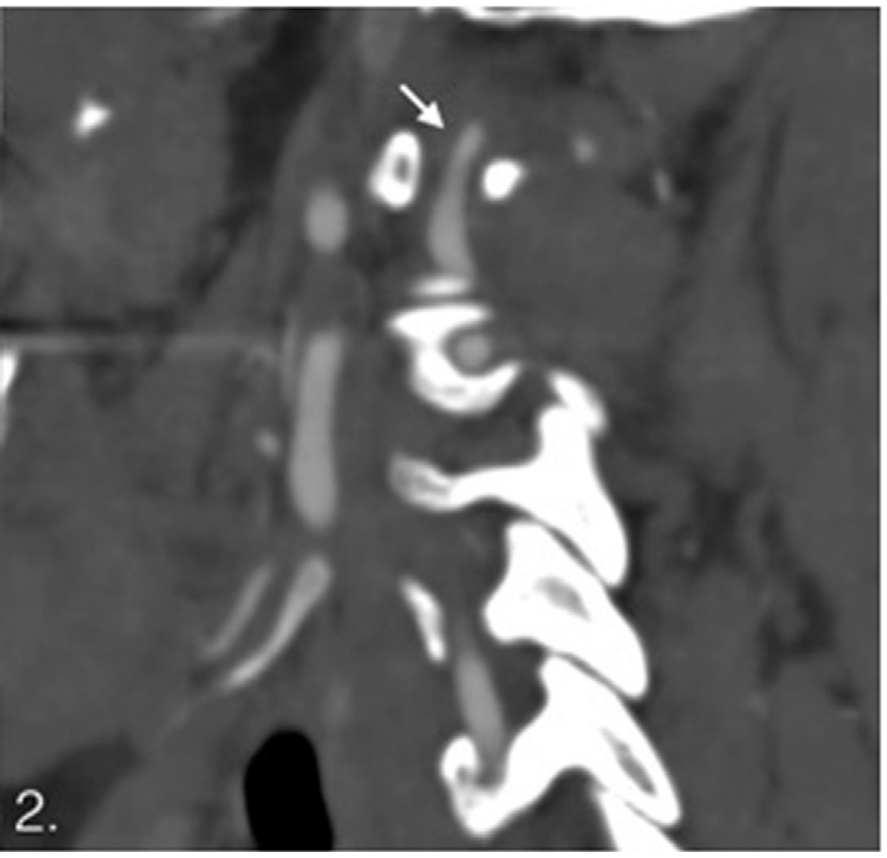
Sagittal computed tomography angiography showing stenosis on V3.
